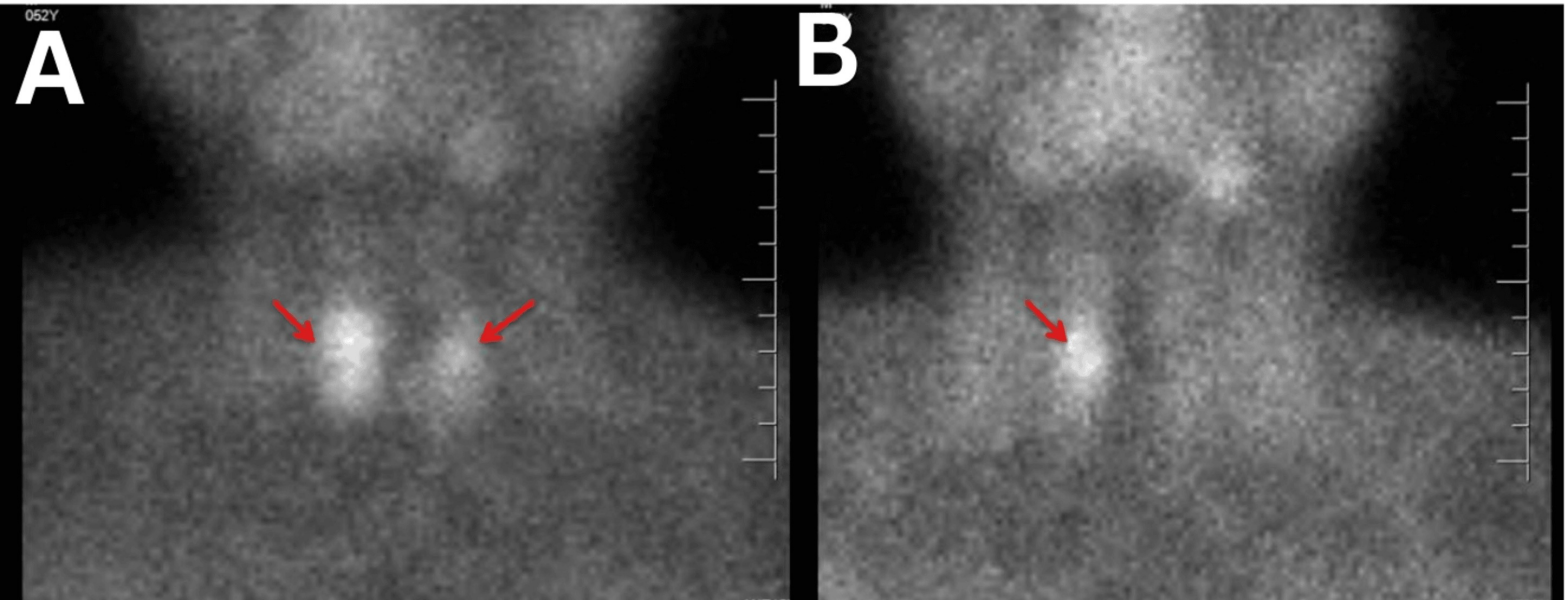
Early (A) and late (B) 99mTc-sestamibi parathyroid scintigraphy images of the neck and mediastinum. In the early phase (A), bilateral uptake is seen just below the thyroid lobes. In the delayed phase (B), only a punctate focus of persistent uptake is noted below the right thyroid lobe (arrow), corresponding to the lesion identified on MRI.
